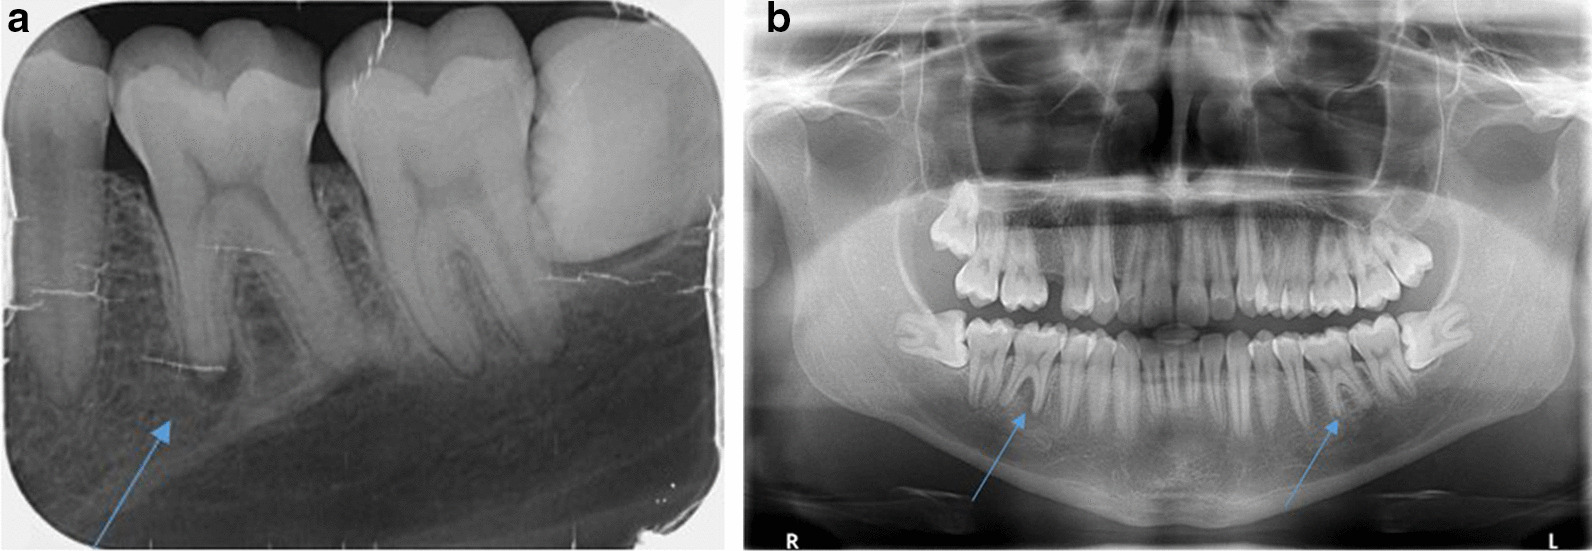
a X-Ray taken in October 2019 by the emergency service dentist. Tooth 36 shows an apical osteolysis. No signs of caries and no fillings are visible. b X-Ray taken in October 2019 by the emergency service dentist. Tooth 36 and 46 show an apical osteolysis. The apical osteolysis is greater on tooth 46. No signs of caries and no fillings are visible
